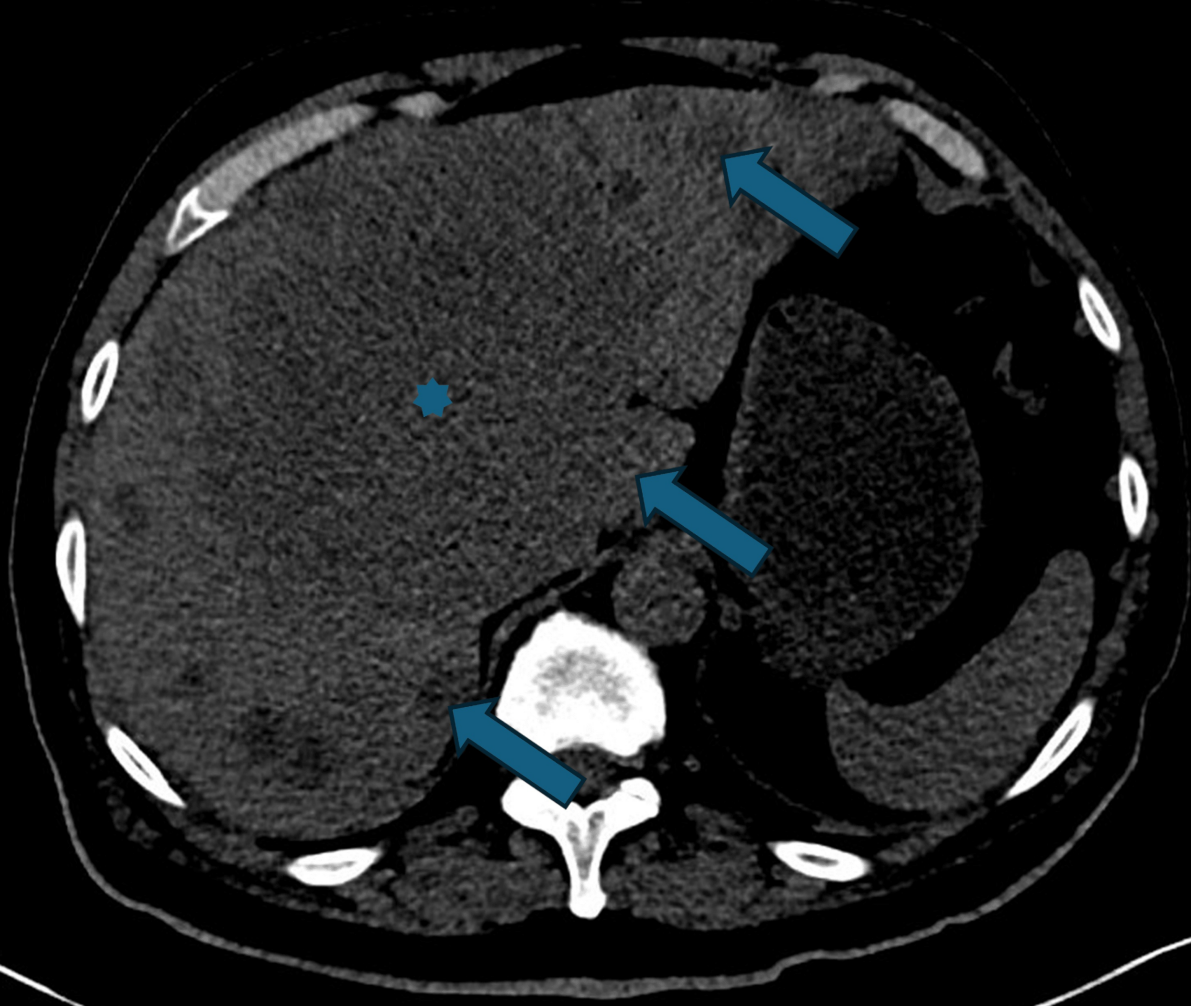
Axial non contrast enhanced abdominal CT scan Multiple liver metastasis (arrows). Central hepatic mass (star)
